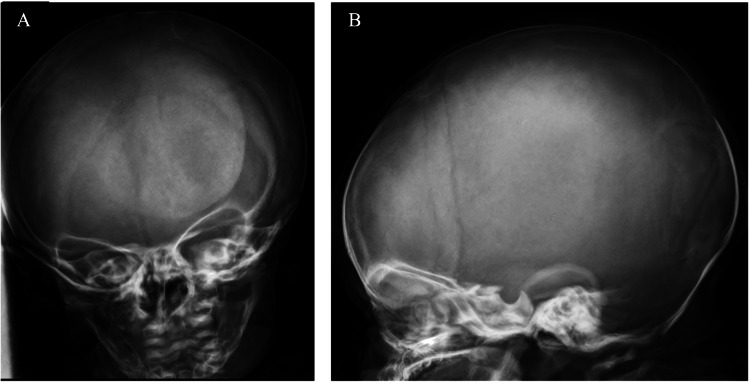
Normal skull X-ray with no evidence of fracture (A: anteroposterior view; B: lateral view)
